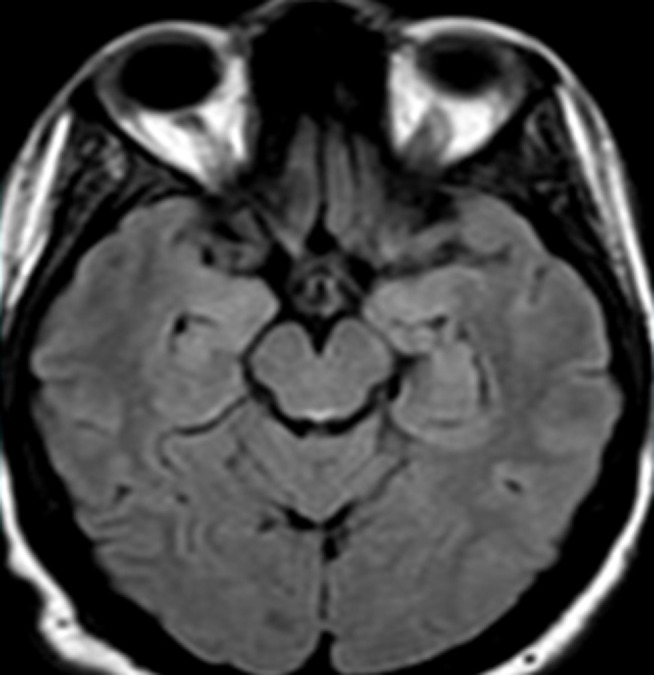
le contrôle IRM réalisé 06 mois plus tard: coupes axiales en séquence FLAIR, note une régression quasi complète des anomalies de signal
